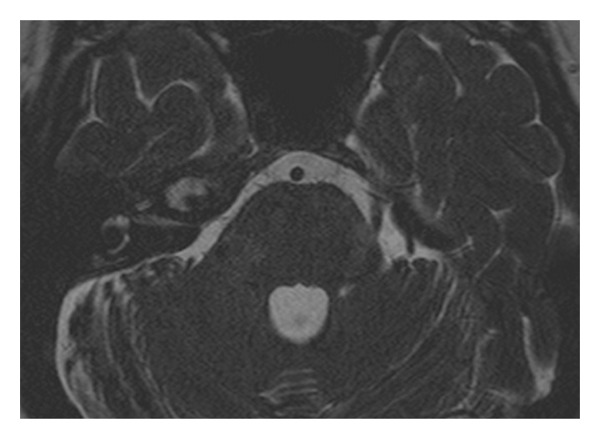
Axial T2-weighted image showes a hyperintense lesion in the right petrous apex.
